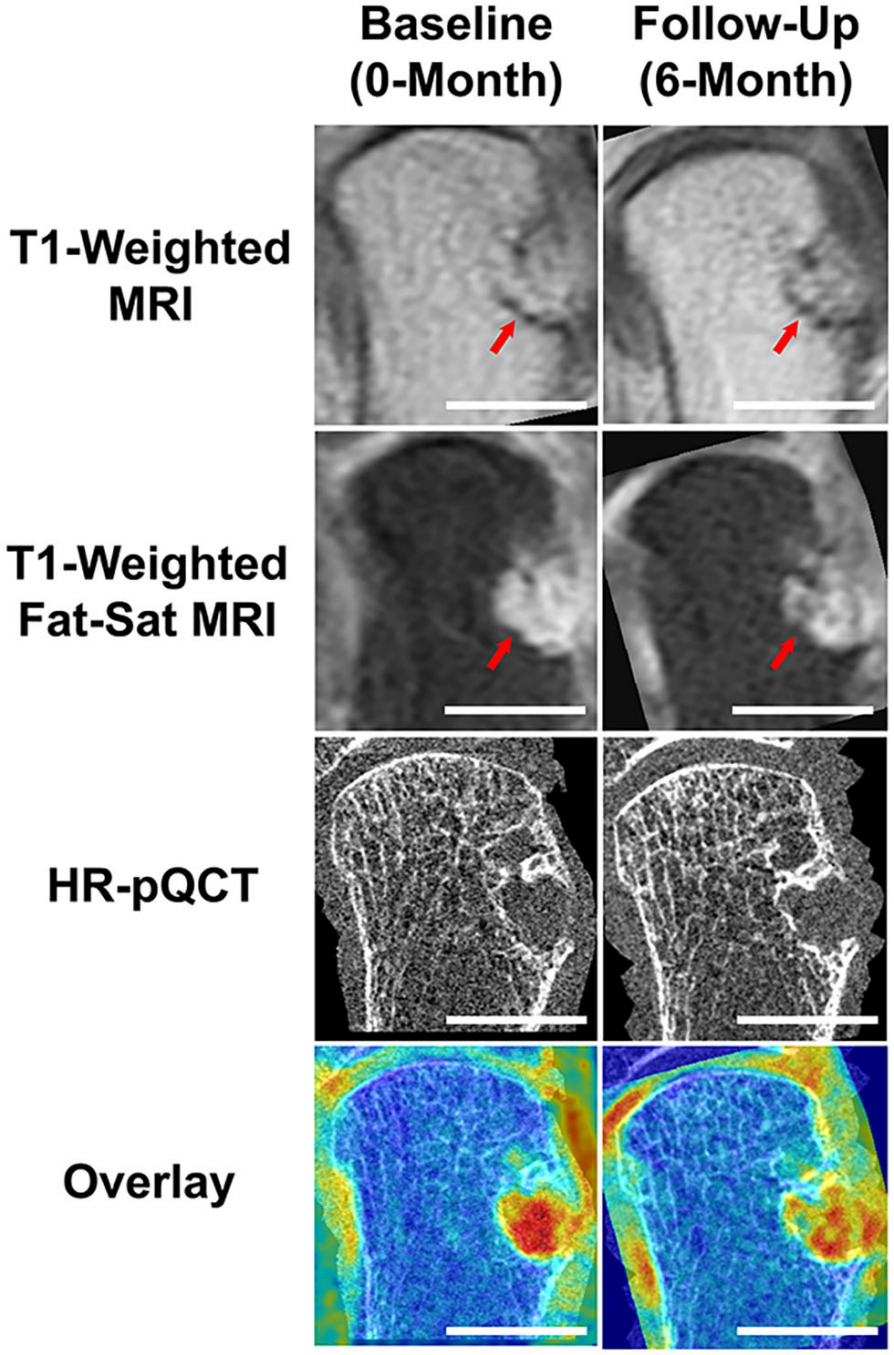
Results demonstrating the benefits of multi-modal imaging. Using MRI and RAMRIS scoring, this RA participant was noted to have a dramatic increase in bone erosion score during their 6-month follow-up, when compared to their baseline score. The post-contrast (Gd) T1-weighted MRI visually displays a larger erosion in the follow-up image (note red arrows). Through accurate image co-registration and overlay, we can observe that the increase in bone erosion score may be due to the differences in positioning during acquisition. While the post-contrast, fat-saturated T1-weighted MRIs display a difference in intensities, the HR-pQCT demonstrates an almost identical erosion size. The Overlay demonstrates the addition of the T1-weighted MRI overlaid with the respective, co-registered HR-pQCT scans. Scalebars represent 1 cm.
